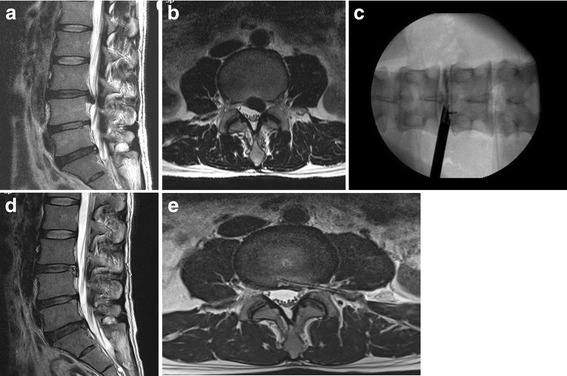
Two months later after second PELD: MR images showing down migrated disc herniation at the L3–4 (a and b). After third PELD (c), MR images showing complete removal of herniated disc and catheter of external drainage in the epidural space (d and e)
